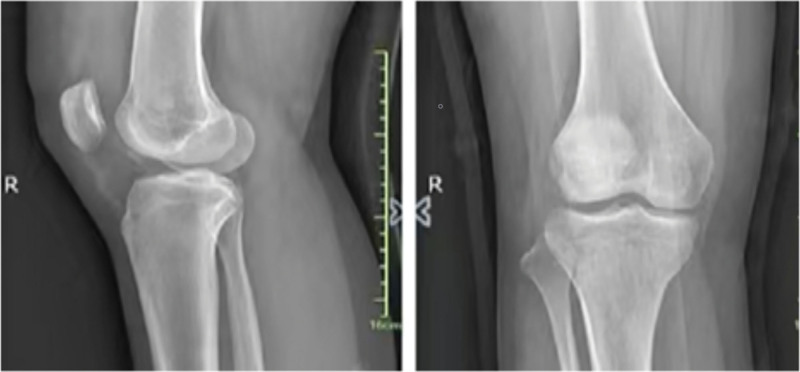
Radiographs of knee joint show loose body in joint.
